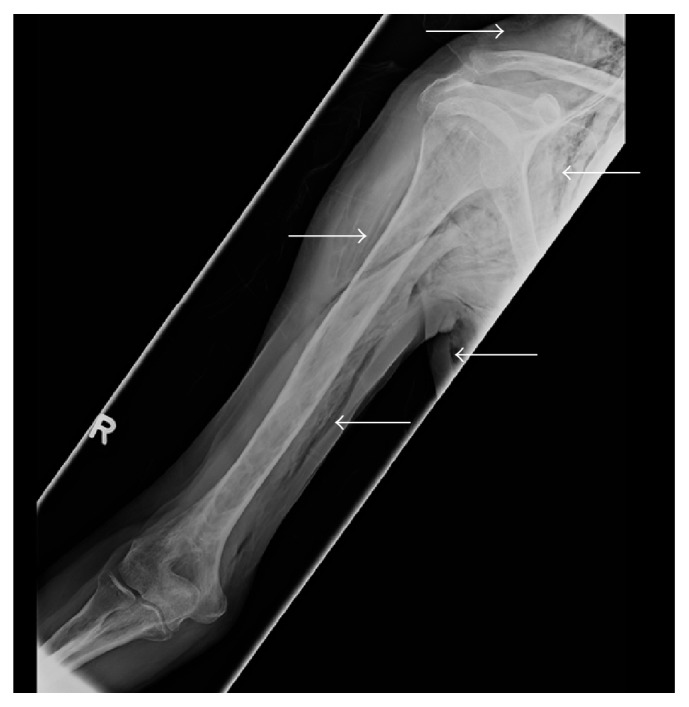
X-ray of the right humeral shaft, indicating emphysema.
